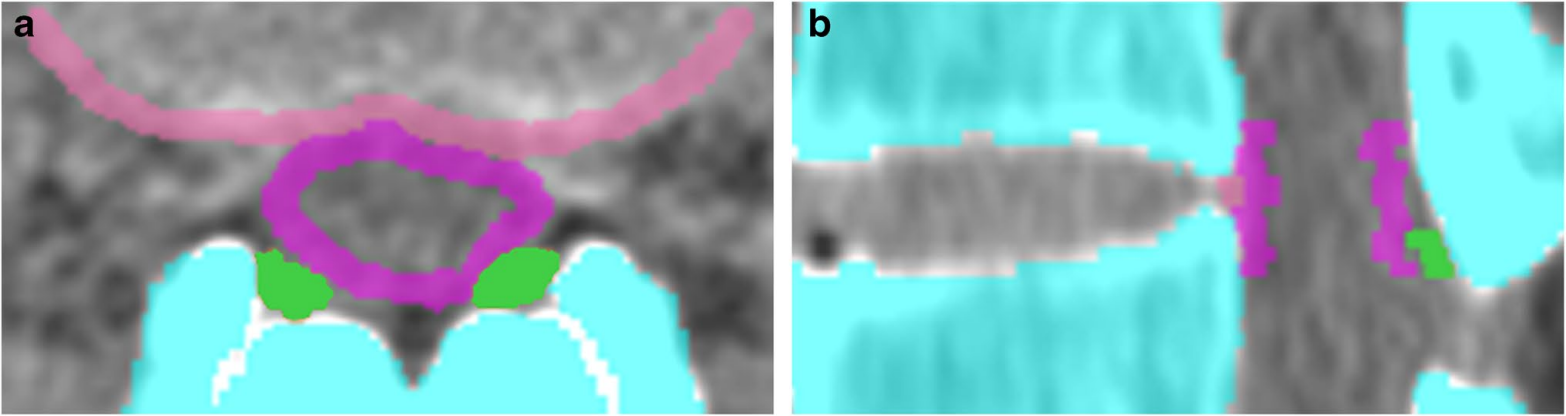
Representative images of the labeling program. The program automatically detects the bone of the lumbar spine (indicated by blue color in the image). Labeling of the dural sac area (pink), disc posterior margin (light pink), and ligamentum flavum (green) on an axial CT scan by the radiologists and students (a). Automatic reformatting of sagittal (b) image by the program
